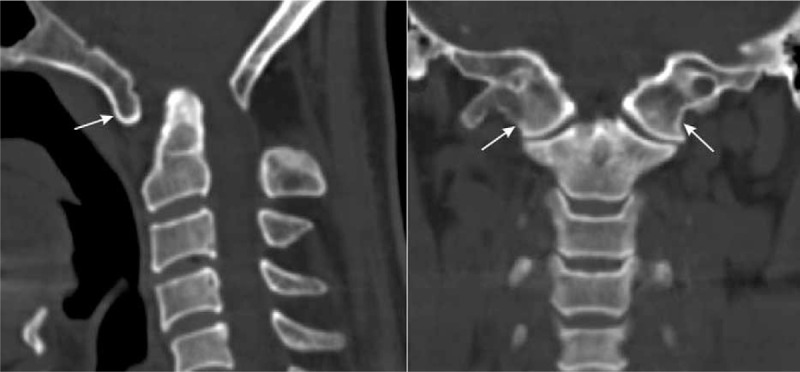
Occipitalization of atlas (arrows) shown in the sagittal plane (left) and the coronal plane (right) on the reconstructed CT images.
